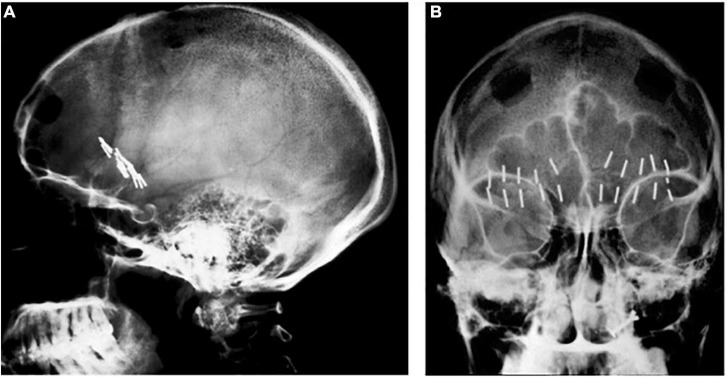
Knight’s early subcaudate tractotomy. (A) Lateral and (B) AP X-rays showing bilateral yttrium seed implants.
